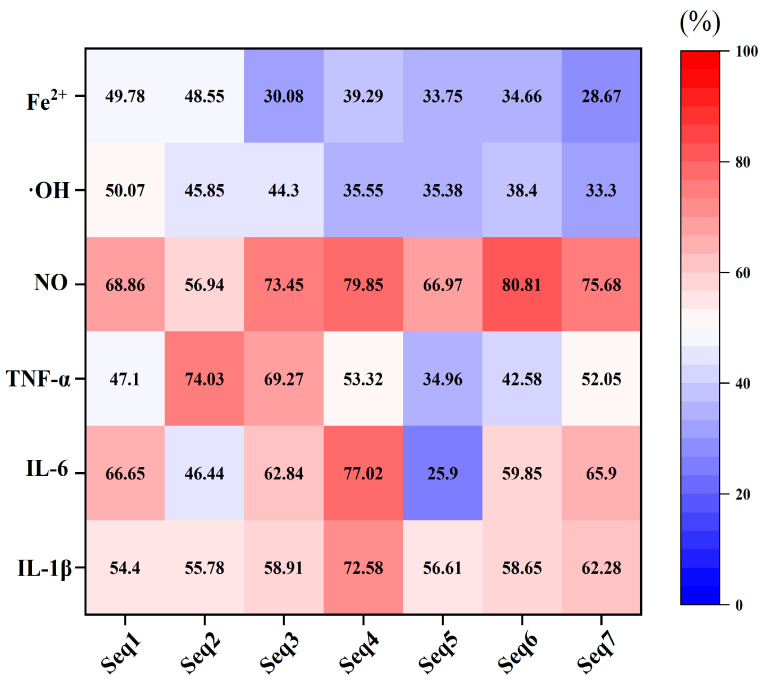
The anti-inflammatory and antioxidant activity in vitro test. Seq1, GGGPGPM; Seq2, GPPGPAGPAG; Seq3, FGFDGDF; Seq4, GPAGPAGPIGPVG; Seq5, AGPAGPAGPAGPR; Seq6, PGPMGPSGPR; Seq7, PAGPAGPIGPV; NO, nitric oxide; TNF-α, tumor necrosis factor-α; IL-6, interleukin 6; IL-1β, interleukin-1 beta; ·OH, hydroxyl radical; Fe2+, ferric ion. The number in each grid presents the mean value of the inhibition rates of inflammatory factors or the antioxidant activity.
